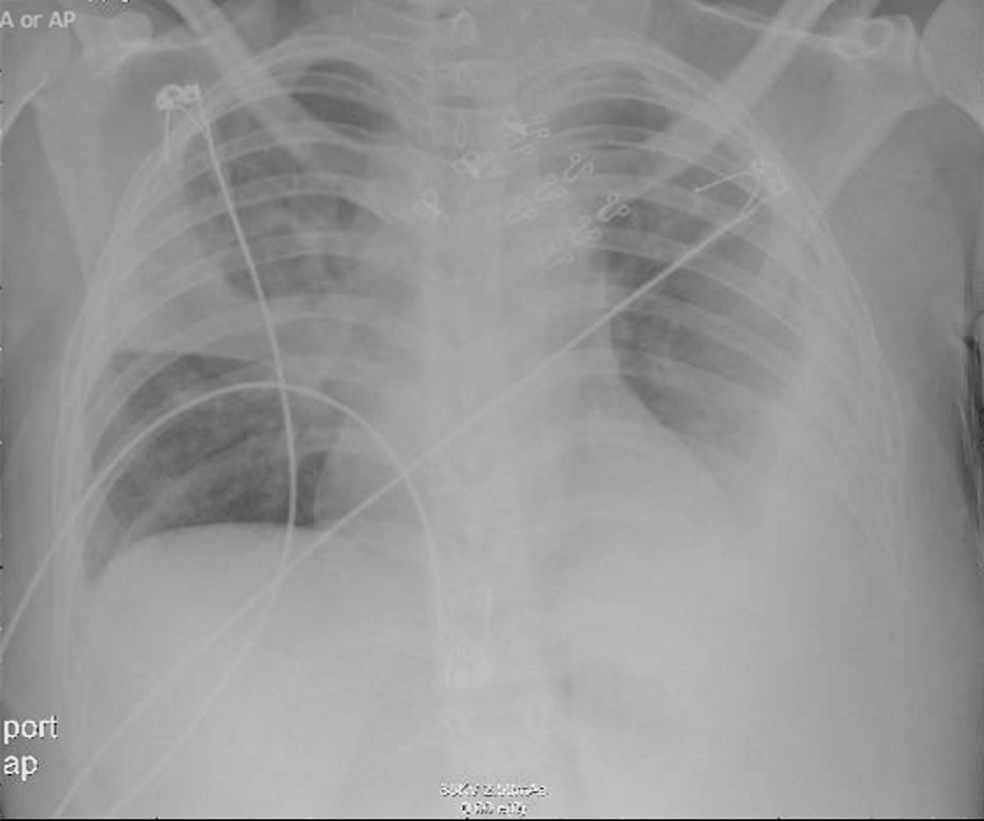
Chest radiograph on admission showing right middle lobe consolidation along with left-sided plural effusion
